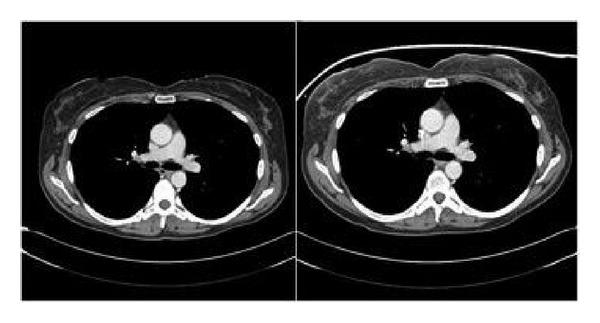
Axial contrast CT scans of the chest. This is an example of a CT scan comparison in which discordance was present between the two readers. The image on the left is a CT with breast shield, while the image on the right is without breast shield. In this case, one reader gave a score 1 while the other gave score 3.
